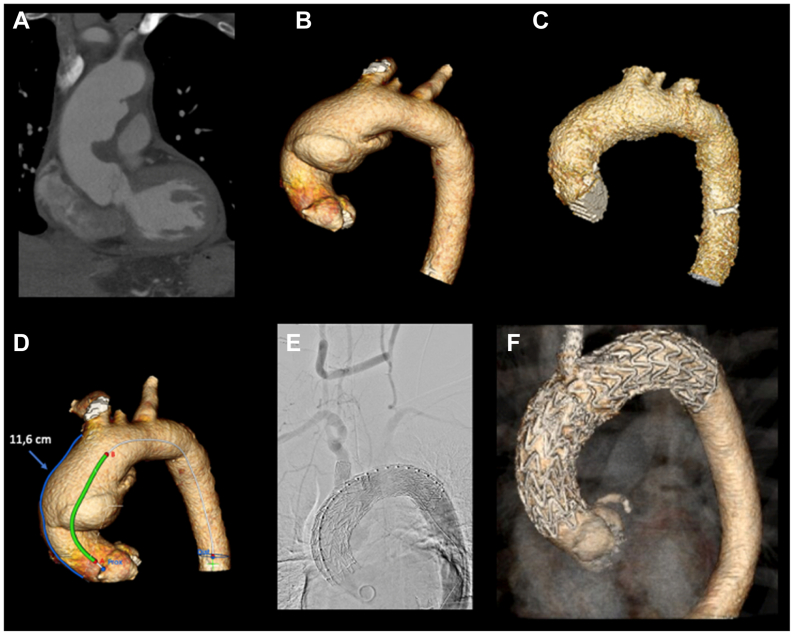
(A) Computed tomography (CT) scan showing a large intimal defect with a partially thrombosed false lumen of the ascending aorta. (B) Three-dimensional reconstruction in of the aortic dissection. (C) Three-dimensional reconstruction of the aorta from a CT scan that was made a few weeks earlier for the follow up of his malignancy. (D) Three-dimensional reconstruction and measurements from the sinotubular junction (STJ) up to the brachiocephalic trunk (BCT). (E) Intraoperative angiogram after stent deployment and patent right to left carotid bypass. (F) Three-dimensional reconstruction of the final result.
